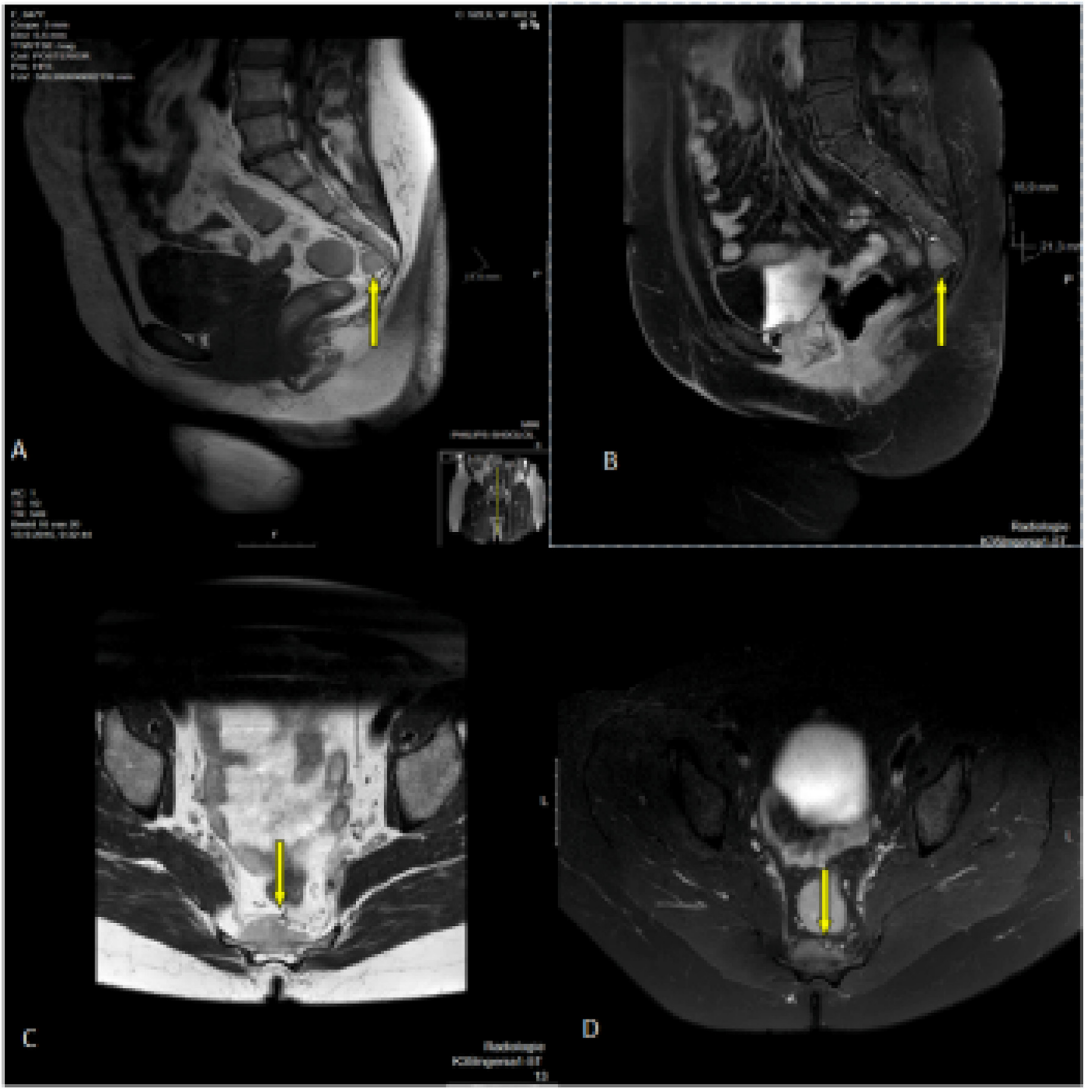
(A and B) Sagittal MRI scans showing how the lesions are in contact with the sacrum where the radiological characteristics of the lesions can be observed; (C and D) Coronal MRI scans showing the lobulated pelvic mass located immediately anterior to the sacrum with no invasion of surrounding structures (arrow).
